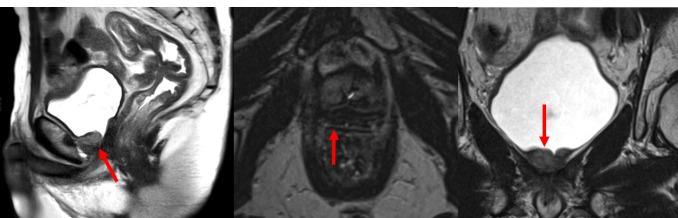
Diagnostic Magnetic Resonance Imaging of a Bulky Recurrence Diagnostic magnetic resonance imaging (MRI) depicting a bulky gross recurrence in the sagittal, axial, and coronal planes. All images shown are T2-weighted turbo spin echo sequence images obtained with a 3.0 Tesla scanner. The red arrows indicate the gross recurrence.
